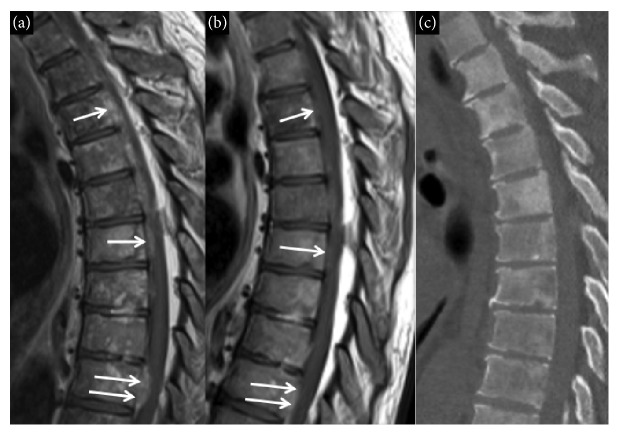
Sagittal contrast-enhanced T1-weighted image of the thoracic spine (a) demonstrates multiple deposits of enhancing soft tissue in the ventral aspect of the epidural space (arrows) consistent with epidural metastatic disease in this patient with prostate cancer. Also note the heterogeneous bone marrow signal consistent with diffuse osseous metastatic disease. A follow-up study obtained just 39 days later (b) demonstrates resolution of epidural metastasis (arrows). Sagittal reconstruction from a thoracic spine CT (c) demonstrates patchy sclerosis in the bone consistent with diffuse osseous metastatic disease.
